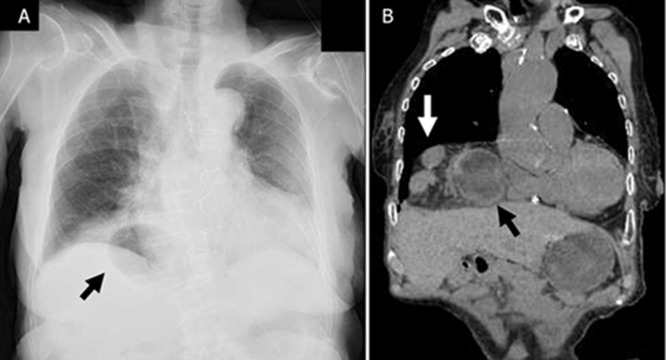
A) preoperative chest radiograph: gastrointestinal gas is observed at the diaphragm (black arrow); B) preoperative computed tomography. Herniation of the stomach (black arrow) and colon (white arrow) is observed
